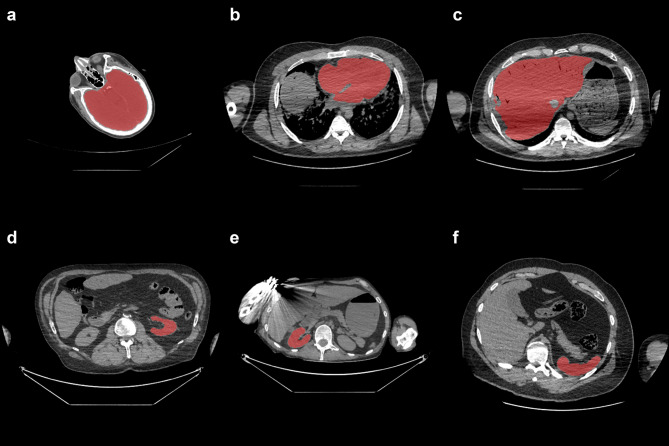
Representative examples of successful AI-based organ segmentations in postmortem CT data. For visualization purposes, single representative CT slices from 3D segmentations are shown for (a) brain, (b) heart, (c) liver, (d) left kidney, (e) right kidney, and (f) spleen. These examples demonstrate accurate anatomical delineation in cases without major decomposition, trauma, or imaging artifacts.
